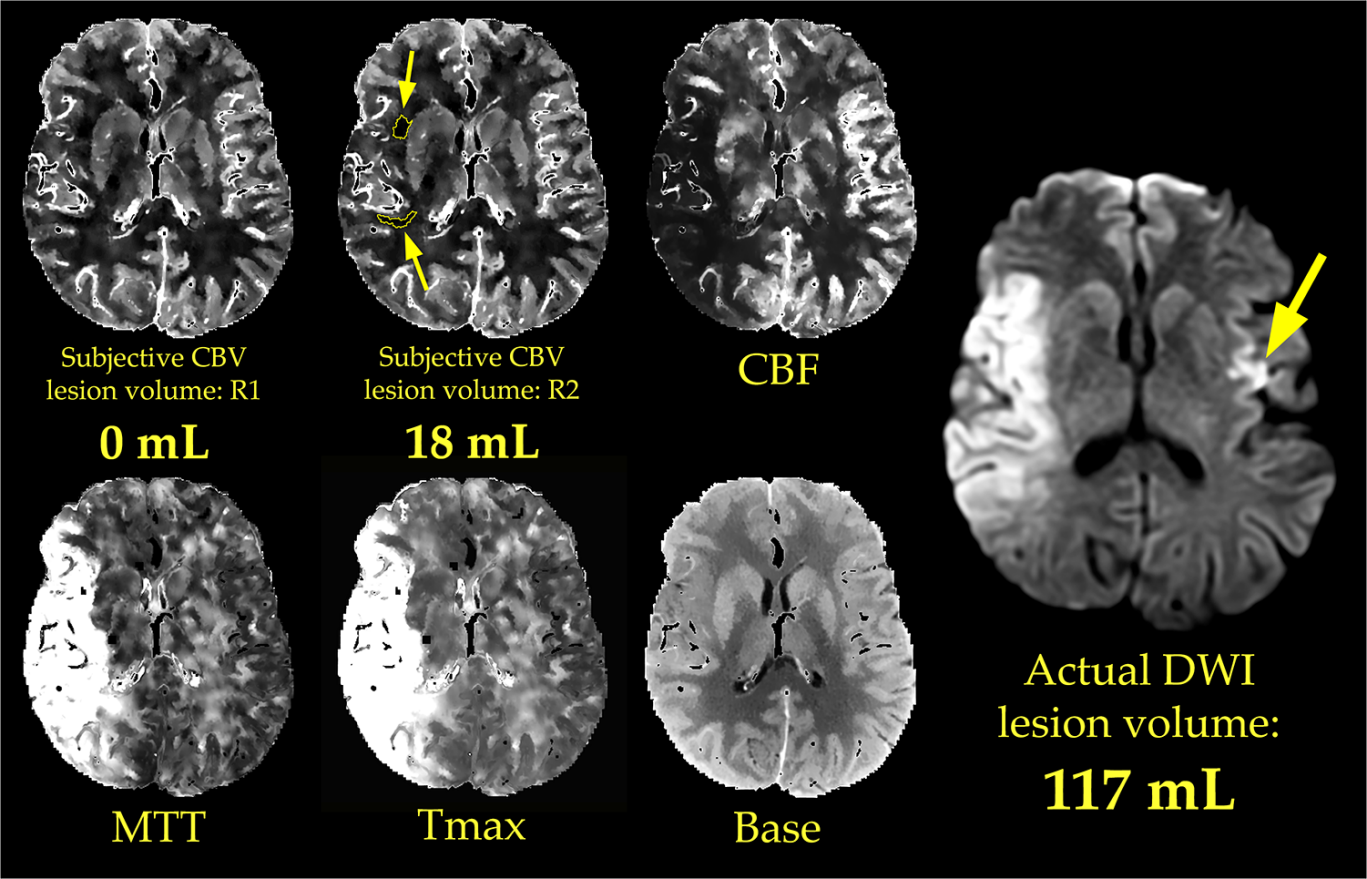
Subjective measurement of CBV lesion volume. Two radiologists (R1 and R2) subjectively outlined regions of abnormally low CBV. In doing so, the radiologists had access to all available CTP images, and to clinical history (in this case, “left face, arm, and leg weakness and left hemianopia”). The patient and slice location are the same as those in Fig 1. R1 did not detect a lesion, i.e. he measured the core lesion volume to be 0 mL. R2 outlined several lesions (yellow outlines and small arrows) with a total volume of 18 mL. R2 failed to identify the presence of infarction in the left cerebral hemisphere (large yellow arrow in the DWI image), which suggested an embolus of central origin rather than one arising from the right internal carotid artery.
